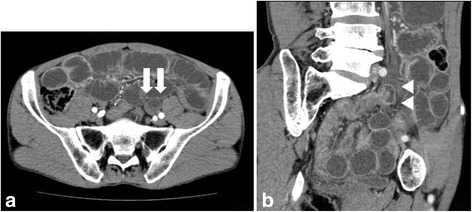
Abdominal computed tomography (CT) scan showing small *bowel obstruction*. a Axial section (white arrows). b Coronal section (white arrowheads)
